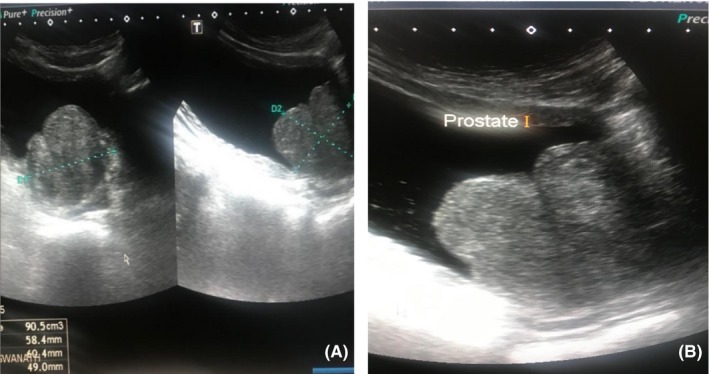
A and B, Transabdominal ultrasound depicting an heterogenous lobular prostate gland measuring 90 cm^3^
